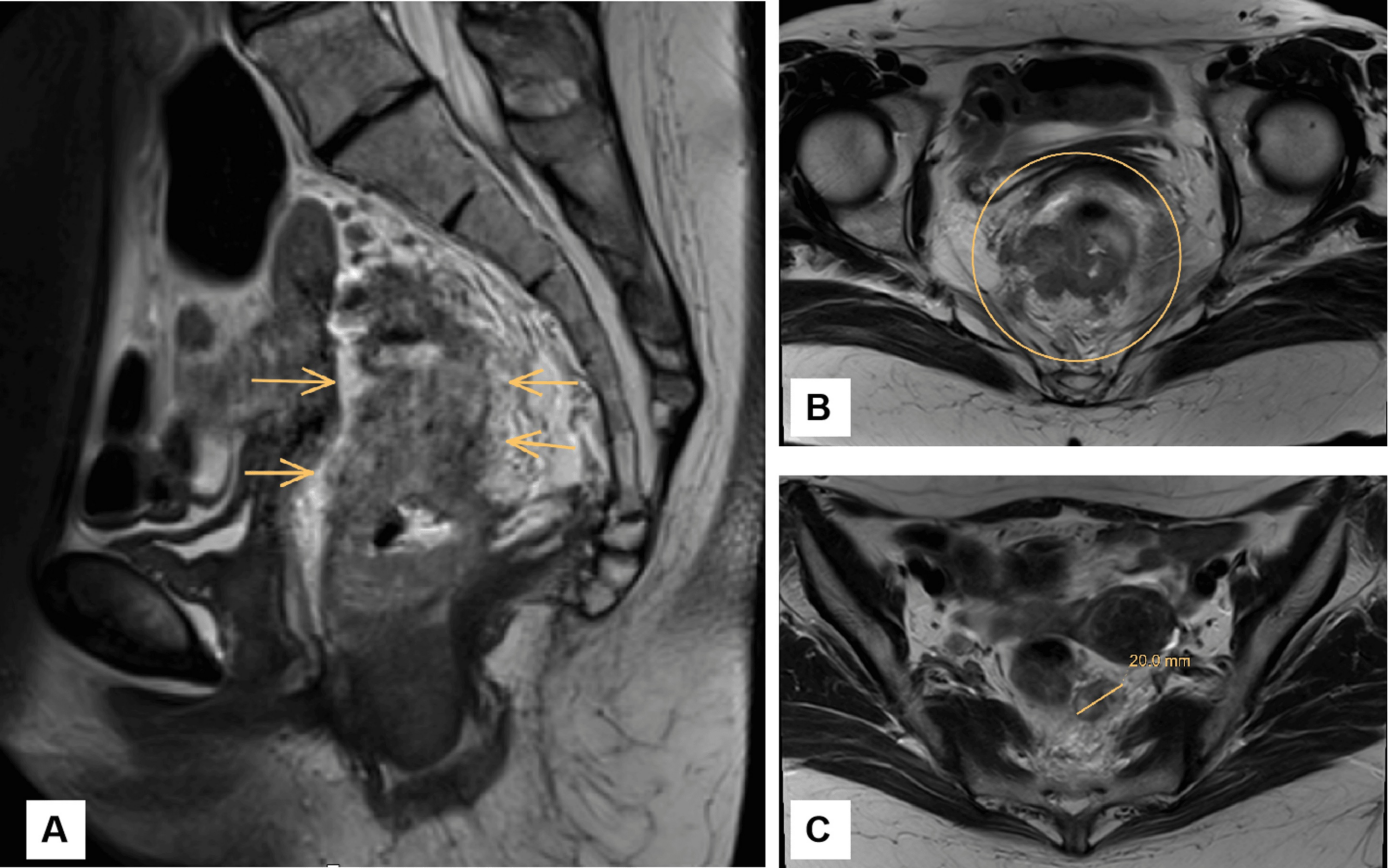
Baseline pelvic MRI obtained before initiation of total neoadjuvant chemoradiotherapy. A. Sagittal T2-weighted pelvic MRI demonstrating marked thickening and irregularity of the rectal wall. The arrows indicate the area of tumor involvement, located approximately 3.3 cm from the anal verge. Stranding and low signal intensity within the perirectal and mesorectal fat are suggestive of extramural tumor extension. B. Axial T2-weighted pelvic MRI showing a heterogeneous mass within the rectal lumen (circled), consistent with rectal adenocarcinoma. The lesion demonstrates irregular wall thickening with intermediate-to-low T2 signal intensity and suggests involvement of the mesorectal fascia. C. Axial T2-weighted MRI showing extramural extension of the rectal tumor into the mesorectal fat, measuring 20.0 mm in depth. This finding is indicative of advanced T3 stage rectal adenocarcinoma.
